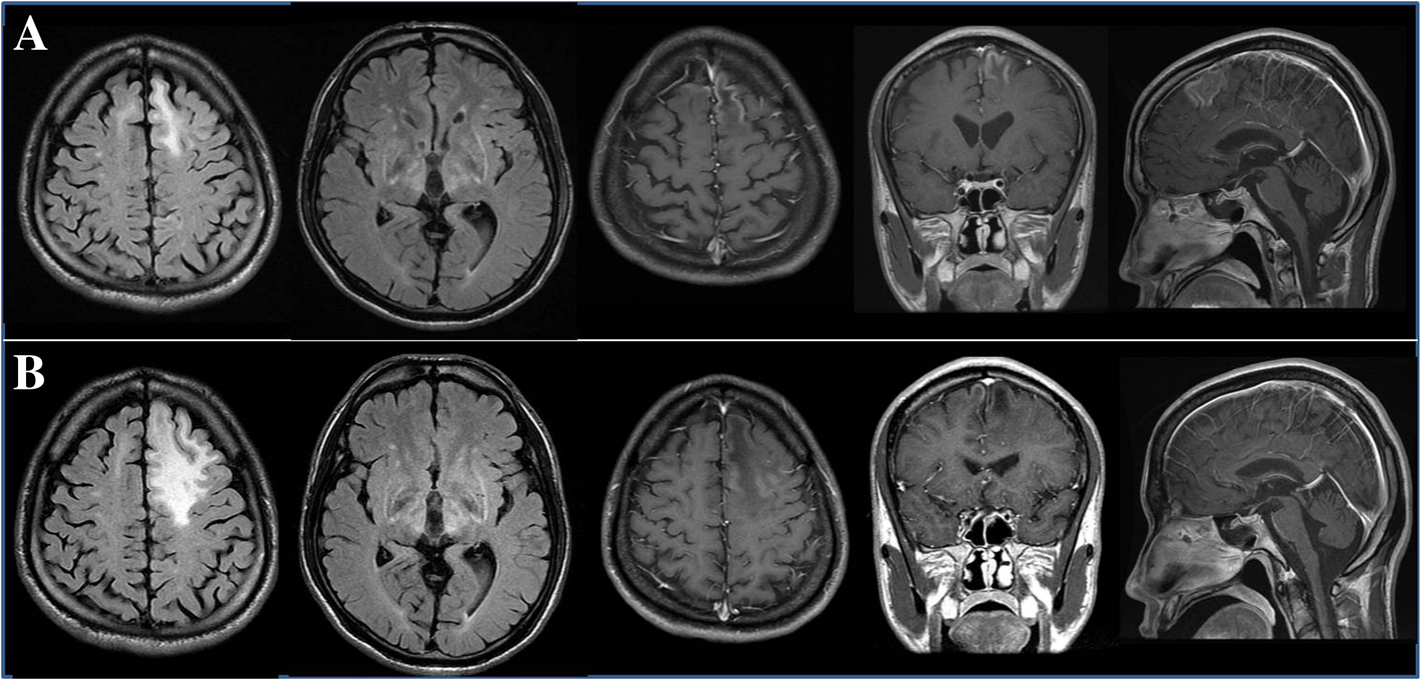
The change of MRI over time (A) MRI before the treatment with antiepileptics after admission shows high signal intensity on both paramedian superior frontal gyri, both basal ganglia and thalamus in the FLARE image, and cortical enhancement on the left superior frontal gyrus in the T1 enhanced image. (B) Cortical enhancement of left superior frontal gyrus resolved at 1 month after treatment with the antiepileptics.
